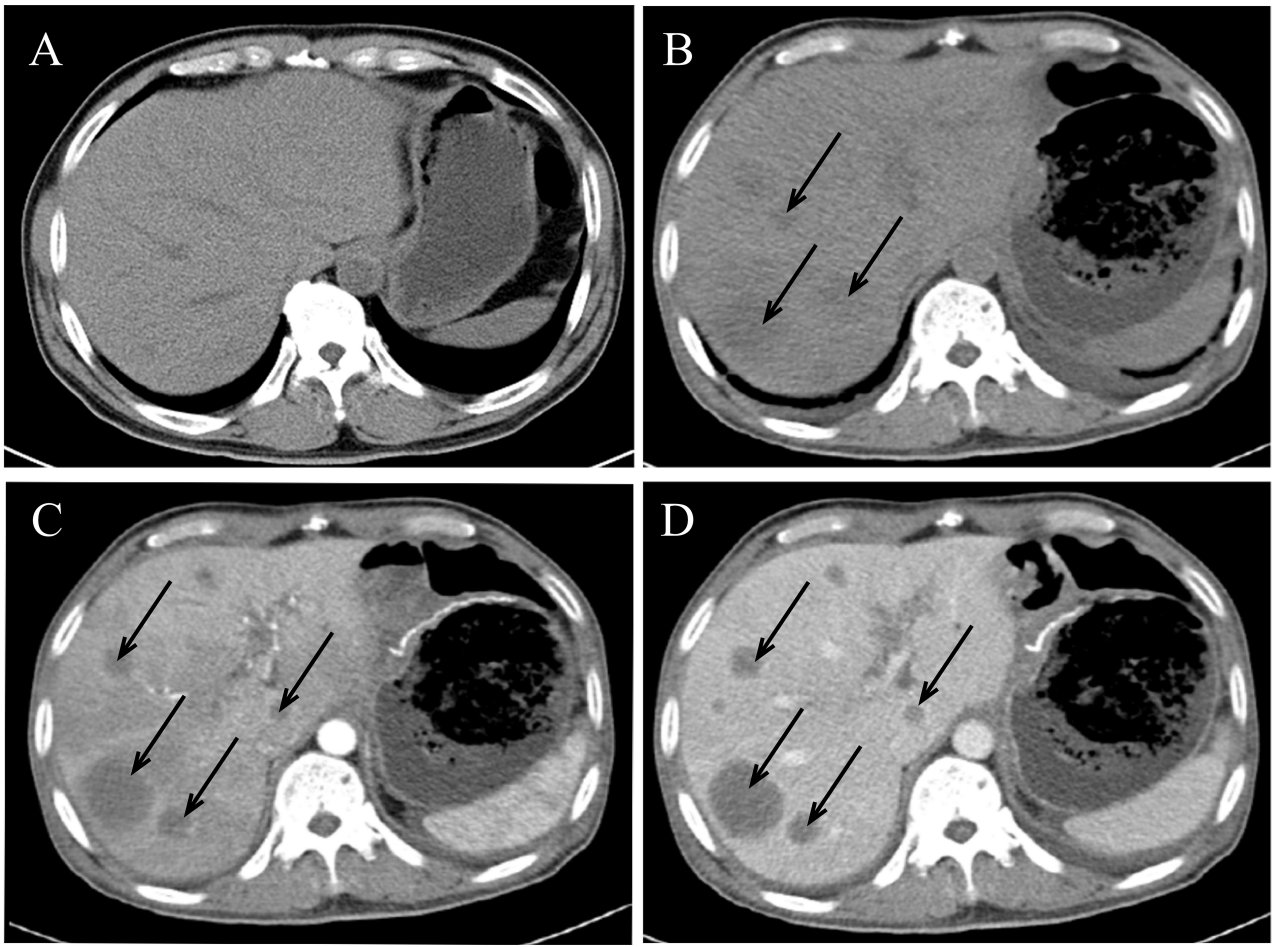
The pre- and post-operative CT findings. (A) The pre-operative CT scan shows no evidence of liver metastases. (B–D) The post-operative CT scans show the presence of multiple liver lesions consistent with metastatic disease (black arrows).
